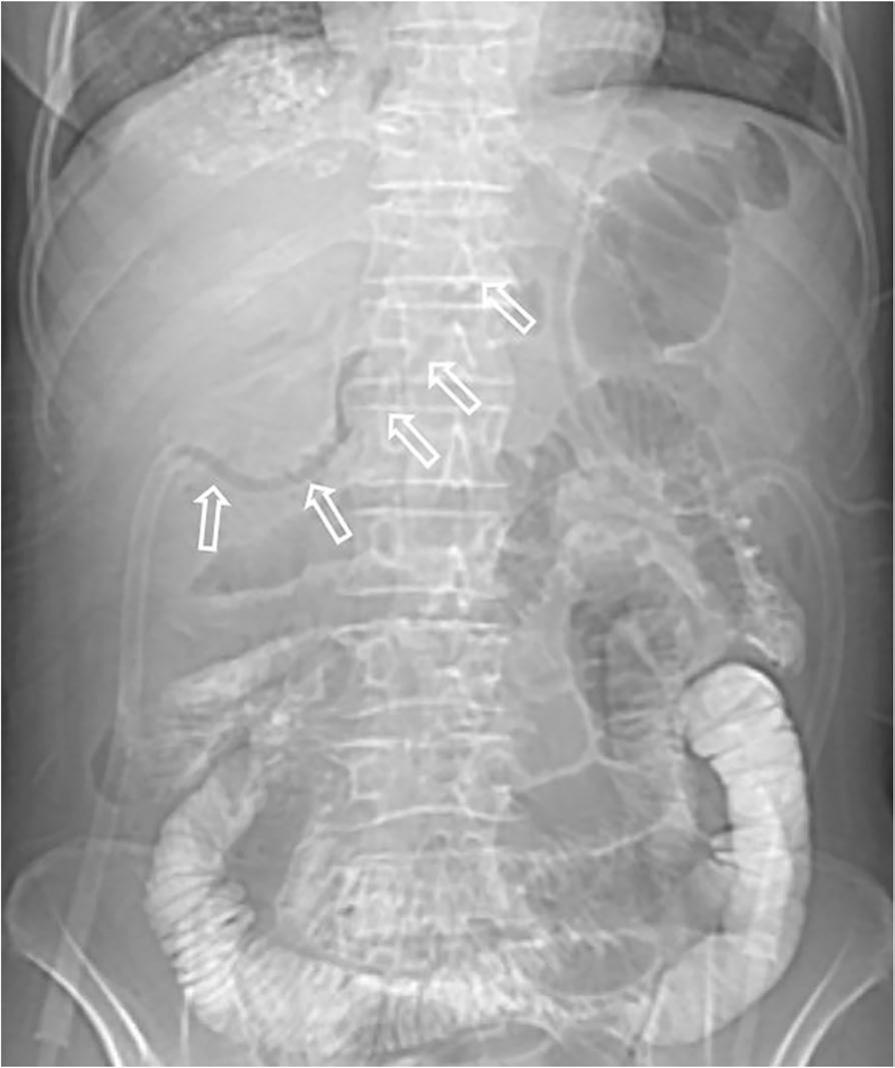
Computed tomography showed that the previous abdominal drainage tube is long and curved, which is difficult to be exchanged for triple-cavity tube
